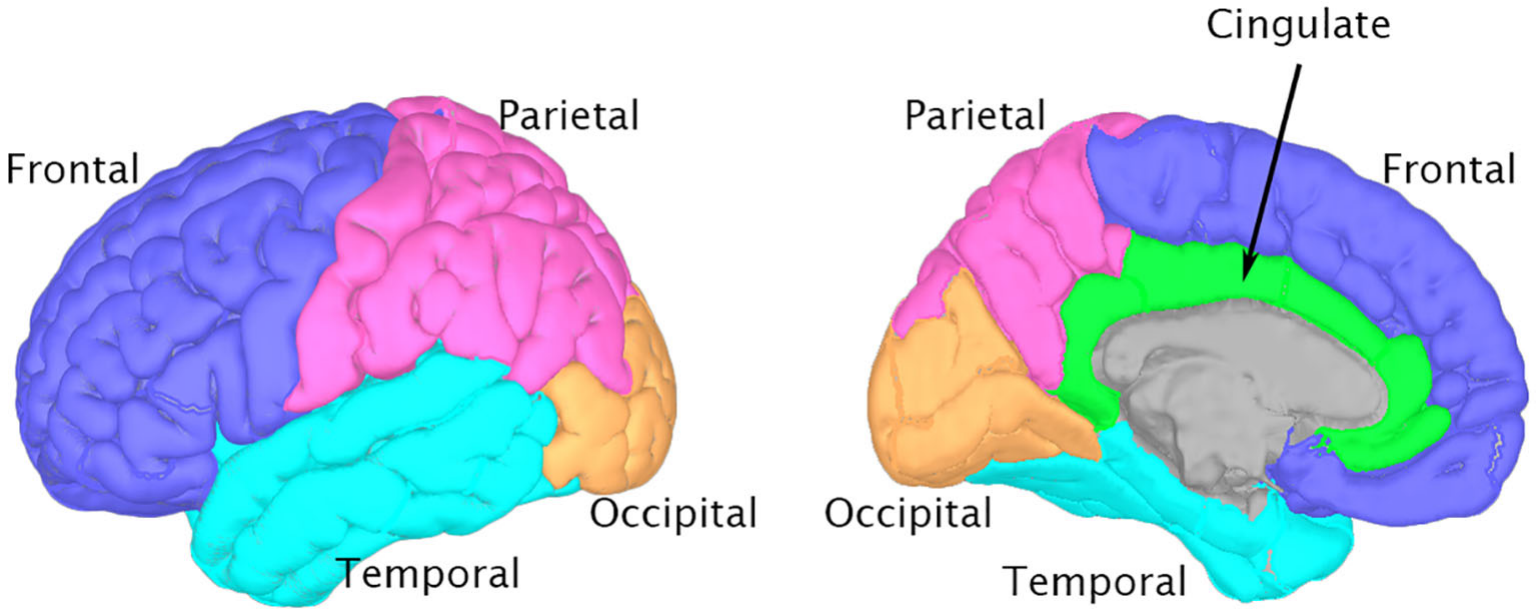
Brain regions for peak alpha frequency Analysis This figure depicts the major brain regions analyzed for peak alpha frequency, based on the Desikan-Killiany atlas. 3D brain models were generated using Brainstorm software (73) and the ICBM152 MRI atlas (74, 75). Left panel: Lateral view highlighting Frontal (blue), Parietal (pink), Temporal (cyan), and Occipital (orange) lobes. Right panel: Medial view showing the Cingulate cortex (green) alongside other visible regions.
